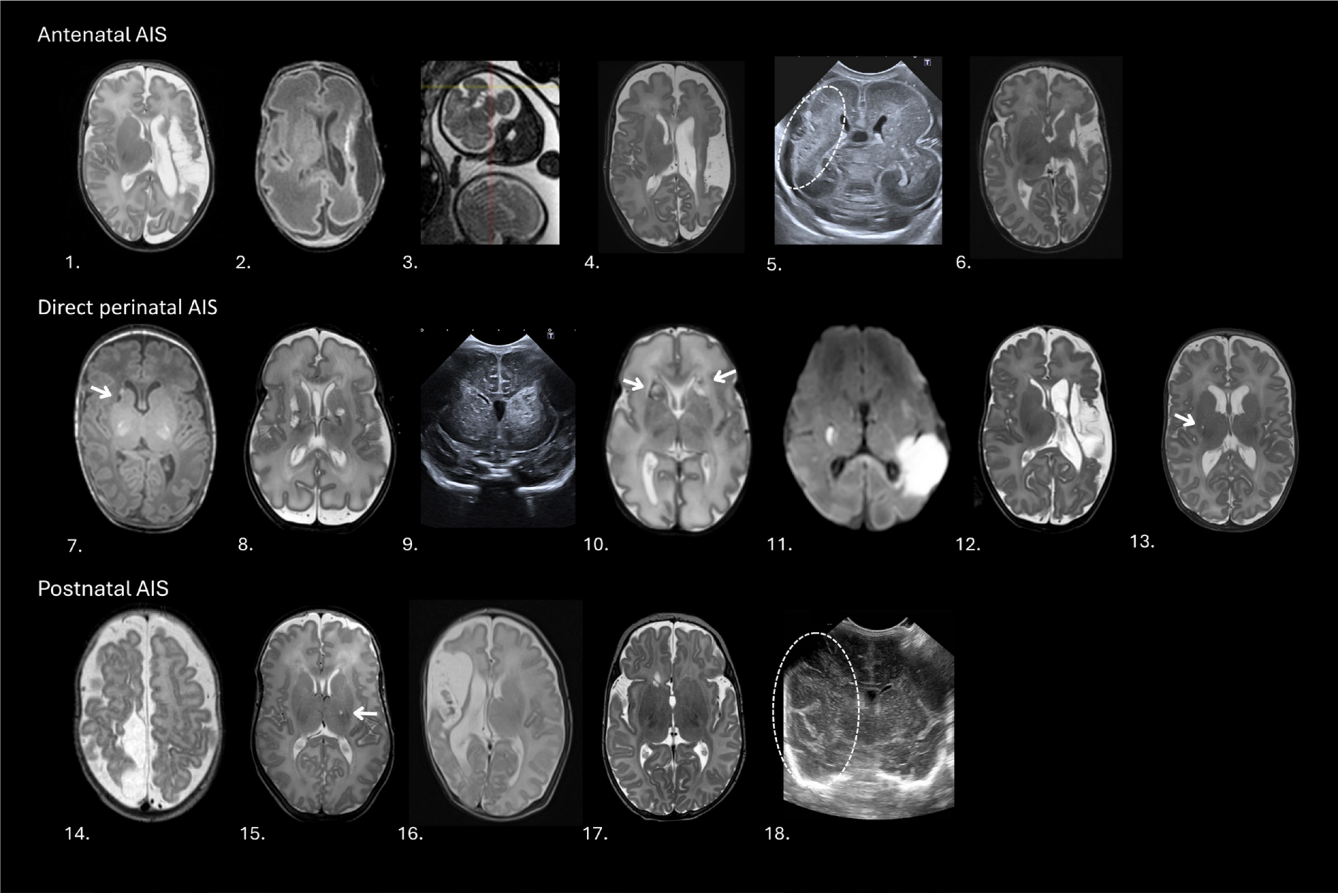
Overview of all stroke patterns on neuroimaging, divided by presumed timing of perinatal arterial ischemic stroke (PAIS). All magnetic resonance imaging (MRI) scans were performed at term-equivalent age, except for twin 3, who experienced a fetal stroke, leading to cord coagulation at 28 weeks’ gestation, and twin 5, who had an acute perimortem twin-to-twin transfusion syndrome following single fetal demise, with an abnormal scan at 24 weeks’ gestation. Both twins died in the neonatal period and therefore did not reach term-equivalent age to perform an MRI. Twin 11 underwent diffusion-weighted imaging in the first week after birth, showing diffusion restriction in both stroke territories.
